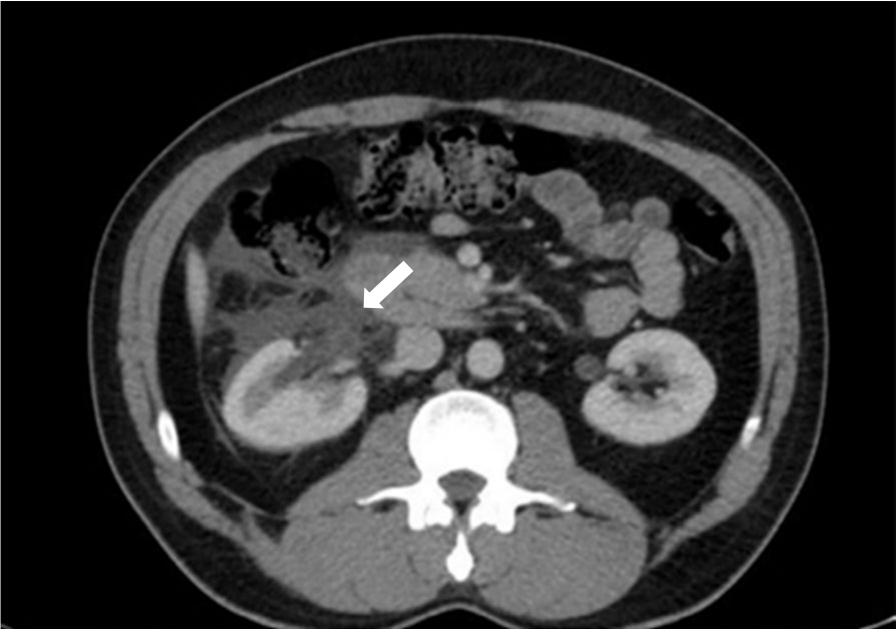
Abdominopelvic CT scan with contrast showing renal pelvis rupture
